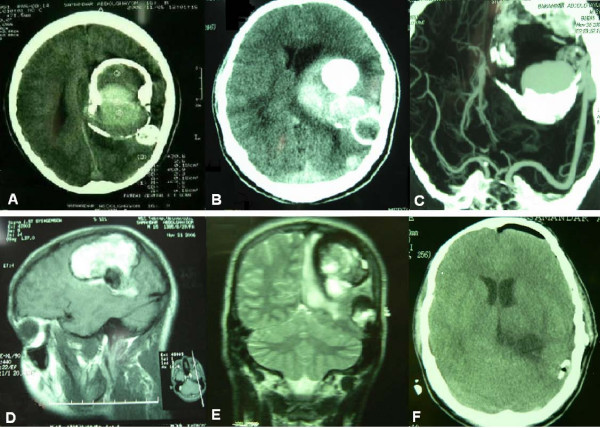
Noncontrast computed tomography showing a huge calcified egg-shaped mass. With a small adjacent calcified lesion (A) and a new episode of hemorrhage (B). CT-Angiography of the lesion (C). T1-weighted parasagittal (D) and T2-weighted coronal (E) magnetic resonance images reveal large mass lesion with signal void rim indicative of calcification and central hypersignal intensity due to met-hemoglobin deposition. Post-operative brain CT scan (F).
